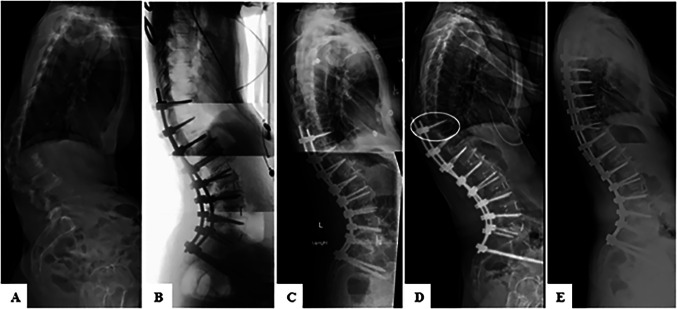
A, Lateral preoperative spine radiograph. B, Intraoperative radiograph. C, First upright radiograph. D, First radiograph with fracture present. E, Postrevision radiograph with an altered screw trajectory at a prior fracture location.
